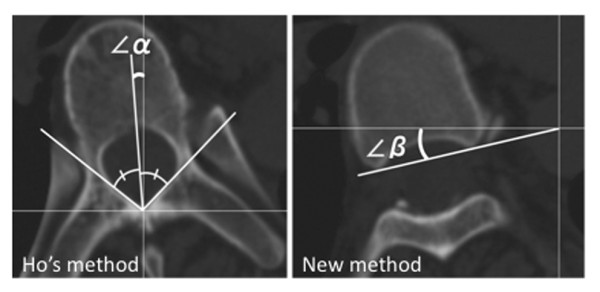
CT measurement methods. Measurement of spinal rotation by Ho's method and by the new method are shown. The inner lamina surface is the anatomical landmark of Ho's method (angle α). The posterior vertebral body beneath the pedicle is the anatomical landmark for the new method (angle β).
